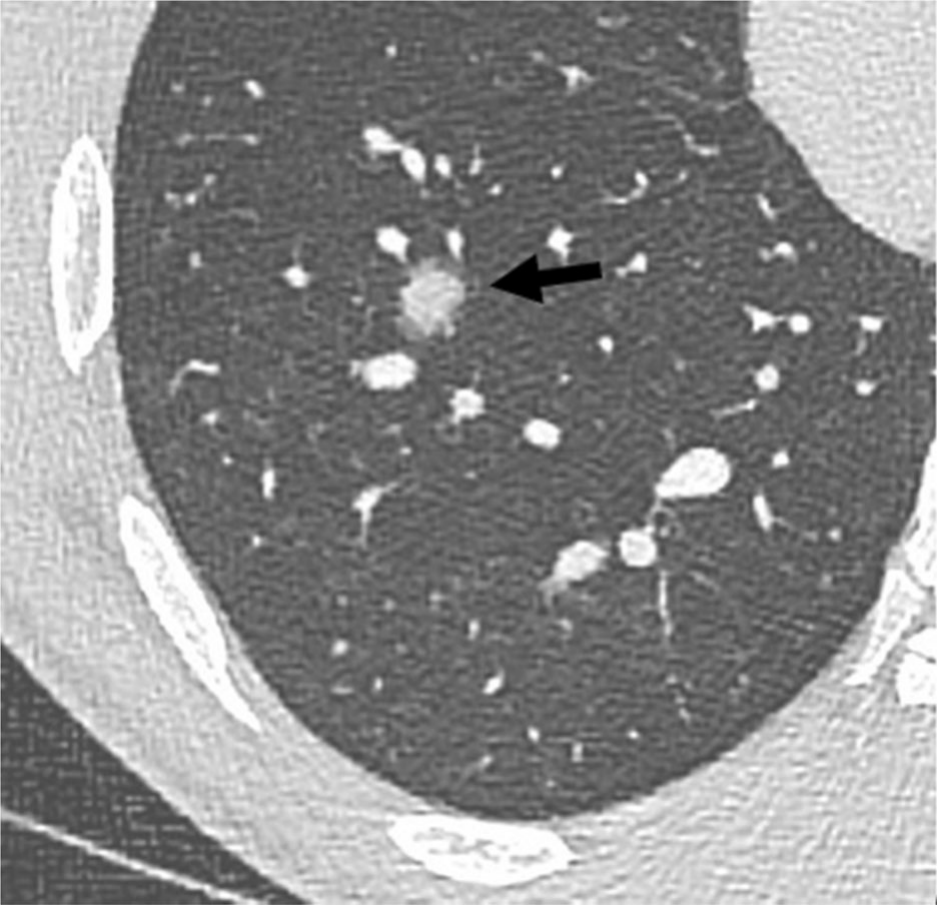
Post contrast axial CT chest with limited HRCT reformats of a 24‐year‐old male patient with GPA presenting with haemoptysis demonstrates a rim of ground‐glass opacity surrounding a consolidated nodule, known as the ‘halo’ sign (arrow).
